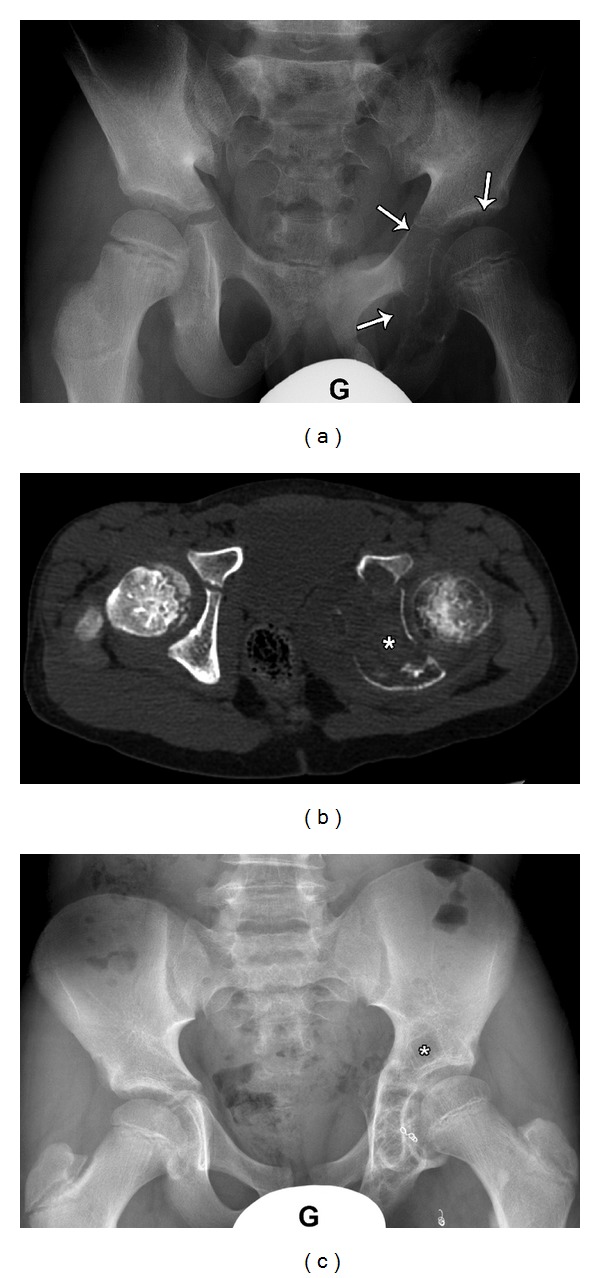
8-year-old male presented with left hip pain, diagnosed as Aneurysmal bone cyst. Plain film (a) demonstrates large expansile lytic lesion (arrows) involving medial left acetabulum extending to the inferior pubic rami (G: gonadal shield). Axial CT scan (b) obtained immediately following curettage and packing with bone allograft showing relatively dense material (asterisk) within the expanded left Ischium. Follow-up plain film (c) five years postsurgical curettage and embolization (note the embolization coils along the medial aspect of the hip) shows healing of the ABS with return to normal contour and course trabeculation. Unfortunately, a focus of recurrence is also noted in the superior acetabulum (asterisk). Local recurrence is not common, but possible.
